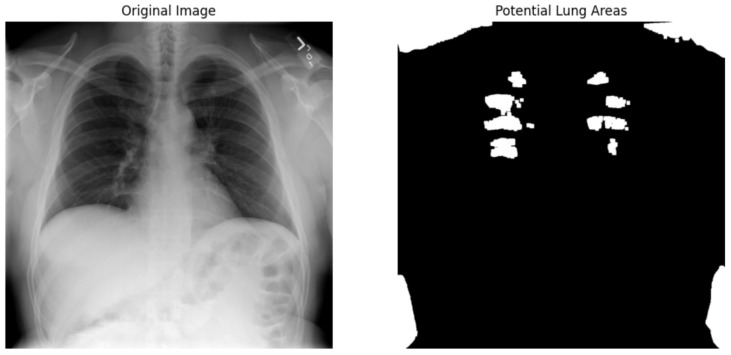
Chest Xray potential area mark on original image to potential lung area.
